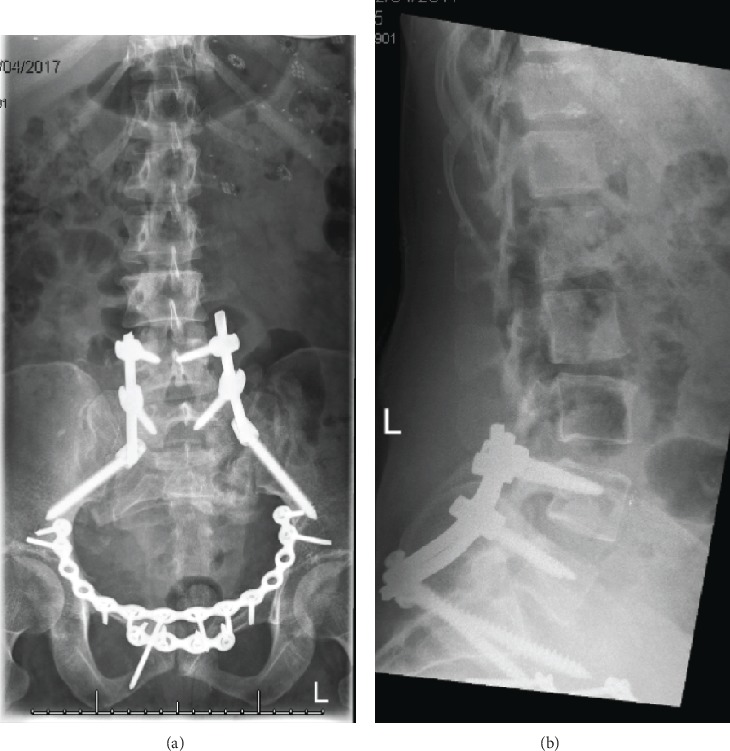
Postoperative pelvic X-rays and lumbosacral X-rays showing bone union in the pelvis and sufficient bone fusion in L4-S1 vertebrae in the antroposterior (a) and lateral views (b), respectively.
